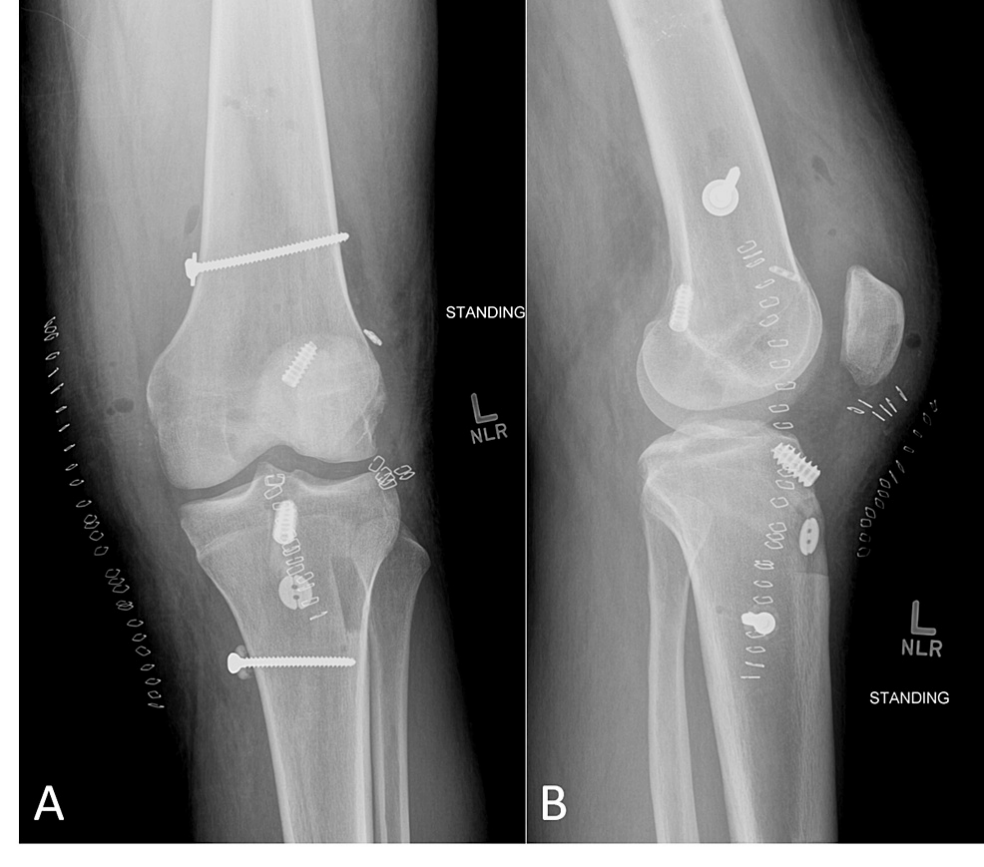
Post-operative radiographic appearance of left knee following anterior cruciate ligament, medial collateral ligament, and posterior cruciate ligament reconstruction (A, B). The instrumentation appears well seated without evidence of convocation.
